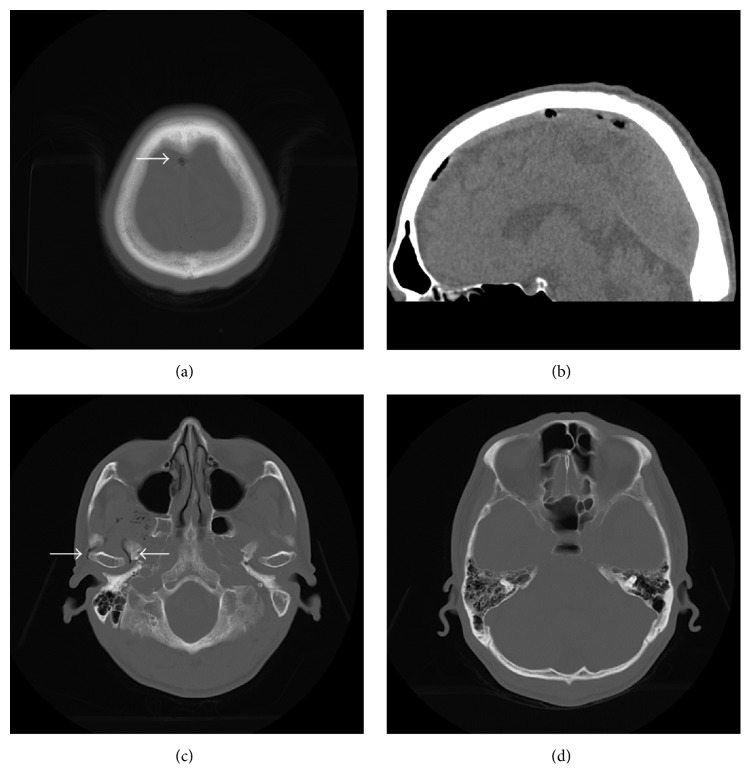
CT scan of the head without contrast: (a) axial cut (bone window) shows air in the superior sagittal sinus (arrow); (b) midsagittal cut of the reconstructed CT (brain window) shows air in the superior sagittal sinus; (c) axial cut (bone window) shows air around the right mandible (arrow); (d) axial cut (bone window) cannot show obvious fractures.
